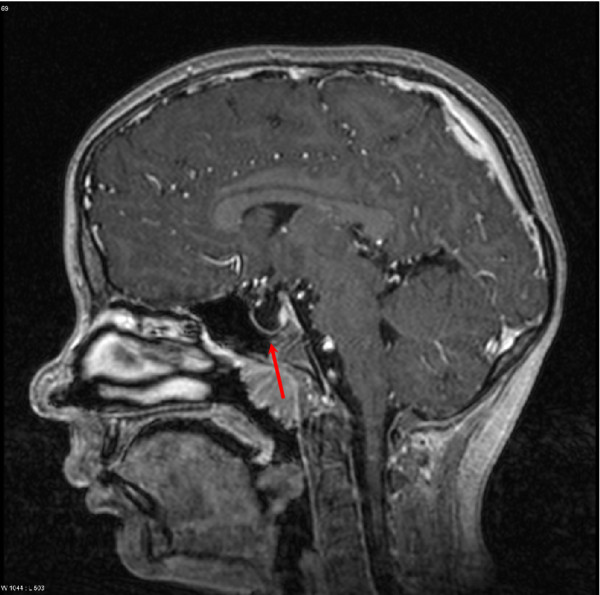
Empty sella. MRI Scan of the brain. Sagittal, T2-weighted. The arrow indicates the empty sella.
